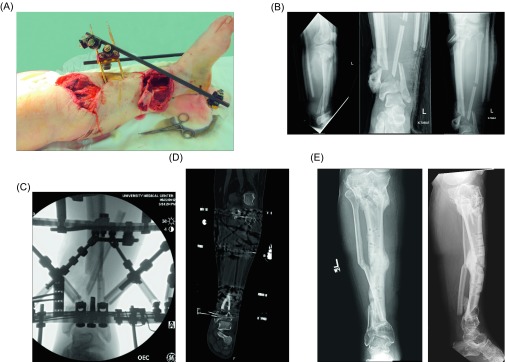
Case example of a 49-year-old male polytrauma who sustained a Gustilo-Anderson grade IIIB open left proximal and distal tibial fractures following airplane crash. (A) Clinical images of large anteromedial wounds over the proximal and distal thirds of the leg with segmental bone loss distally, (B) anteroposterior and lateral injury radiographs demonstrating the aforementioned injuries with associated fibular fracture, (C) initial post-operative fluoroscopic images with Taylor Spatial Frame (TSF) in place, (D) coronal CT renderings of the distal tibial fracture at the time of nonunion (seven months following injury) with Taylor Spatial Frame in place, and (E) final film taken one year following RIA at the time of union and TSF removal.
